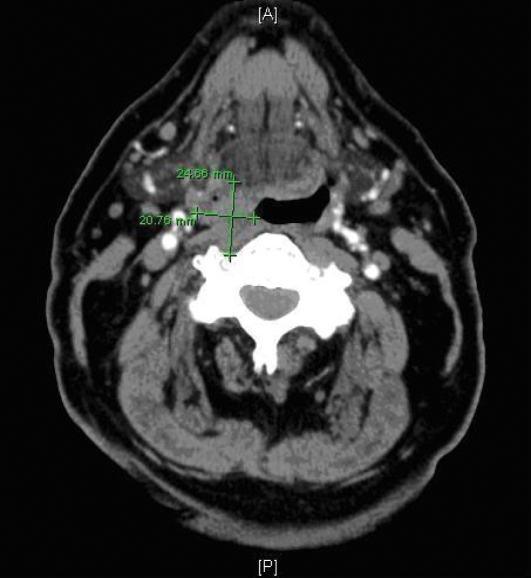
Neck CT with contrast showing a soft density mass in the right hypopharynx lateral wall extending around the lateral pharyngeal wall into the prevertebral soft tissues on the right.
